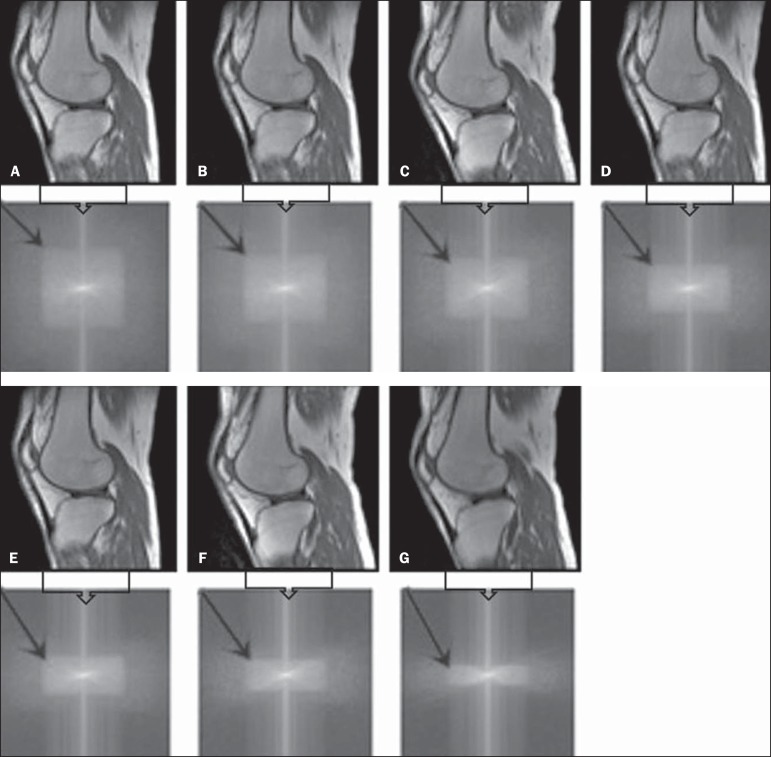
T2-weighted magnetic resonance images with the following ScP variations:
